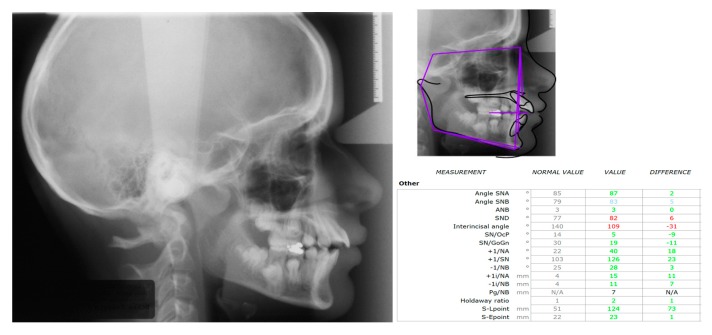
Pretreatment cephalometric radiograph showing a skeletal Class II profile.
